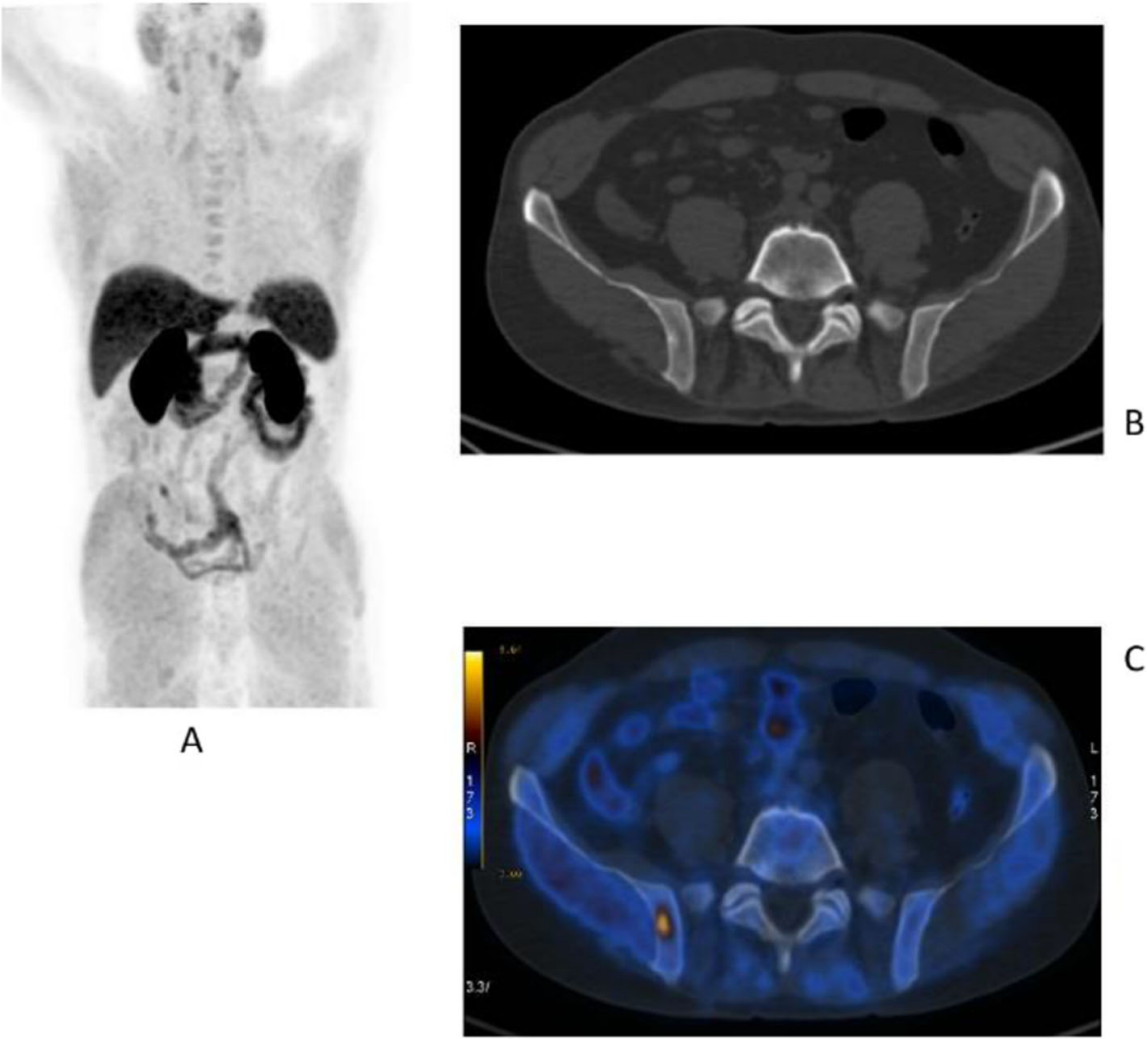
^11^C-choline, biochemical recurrence (BCR). *Clinical history*: 70 y.o. man with prostate cancer, Gleason score 4 + 3, treated with radical prostatectomy as primary treatment; BCR with a TTR 48 months and PSA 0.6 ng/ml PSAdt 5 months. *PET/CT findings*: focal increased uptake seen in the right iliac bone. MIP (a), CT (b), and fused images (c) showed a very small osteoblastic lesion
